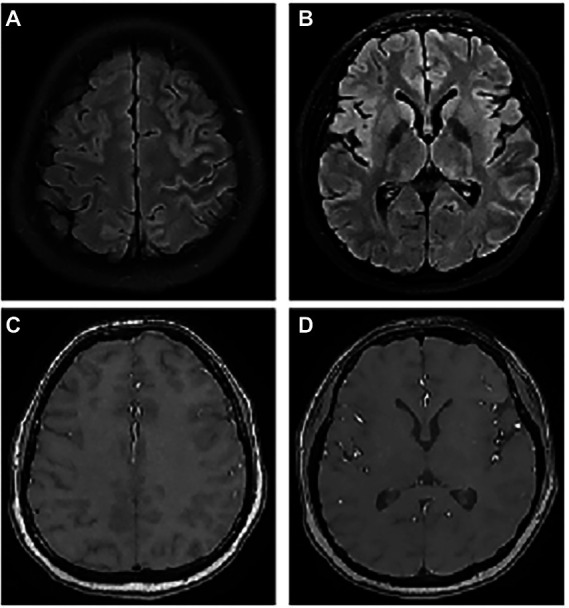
The abnormal MRI was obtained from patient 6. A 23-year-old woman with serum MOG antibodies present with headache only. Axial T2Flair images show hyperintense cortical signals in the left frontal, temporal, and parietal lobes (A,B), but enhancement was normal (C,D).
